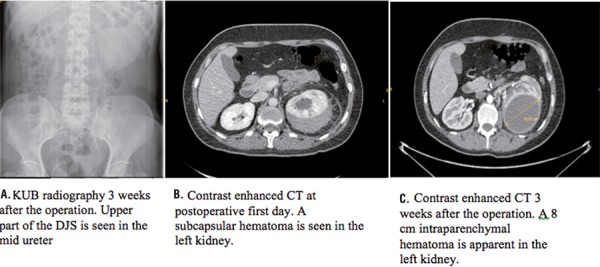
Postoperative KUB radiograph and contrast-enhanced abdomen tomographies.
